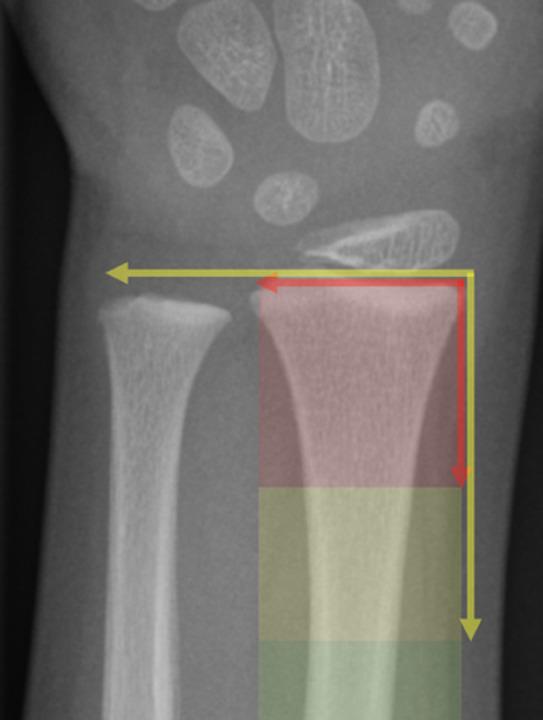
Radiographic definition of metaphyseal (red), diametaphyseal (yellow), and diaphyseal (green) regions of the distal radius based on projection of distal radial width onto the longitudinal axis
